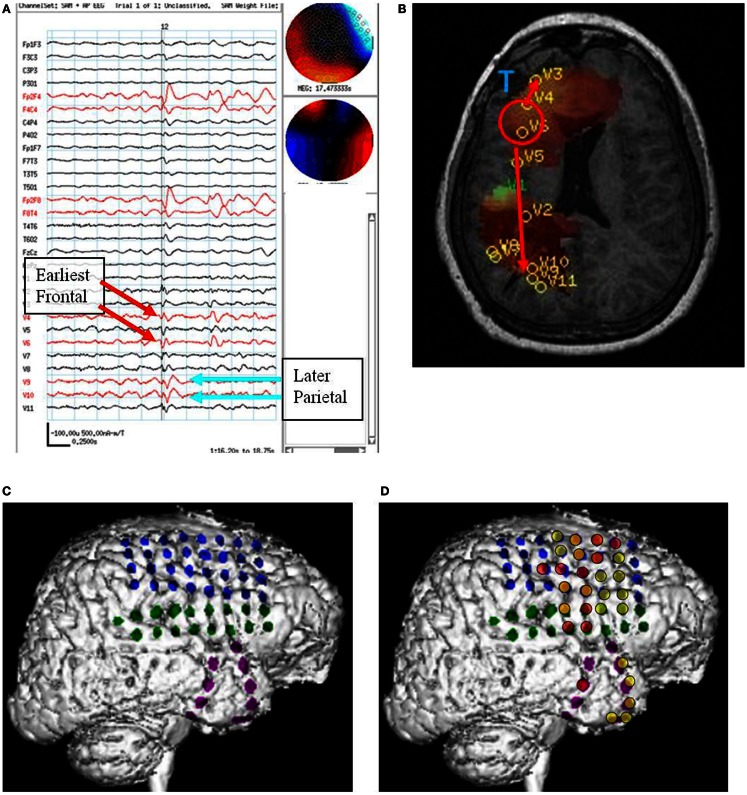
Subject 4. (A) Bipolar AP EEG and 11 virtual sensor channels showing interictal spike in unaveraged signals in bandwidth 3–70 Hz, digitized at 300 Hz. The earliest peaks occurred at V4 and V6 right frontal lobe (red arrows), just posterior to the resection site for tumor, followed 100 ms later by spike peaks at V9 and V10 right parietal lobe (blue arrows). In an expanded timebased view (not shown), V9 and V10 also show smaller amplitude spikes earlier, 20 ms after V4 and V6. In that view, timing of the spike at V3, right frontal lobe, is also 20 ms after V4, V6. (B) Subject’s MRI scan showing tumor resection right frontal lobe (blue T), interictal spike onset at V4, V6 (red circle), and subsequent spike peaks anteriorly at V3 and posteriorly at V9, V10. Red arrows indicate relative timing of peaks. (C) 3D reconstruction of subject’s MRI scan with subdural grids over right frontal and temporal lobe. (D) Summary of electrodes active at or shortly after seizure onset with the most intense red color corresponding to the most active electrodes in superior, mid, and inferior frontal lobe posterior to resection site and also mid temporal.
